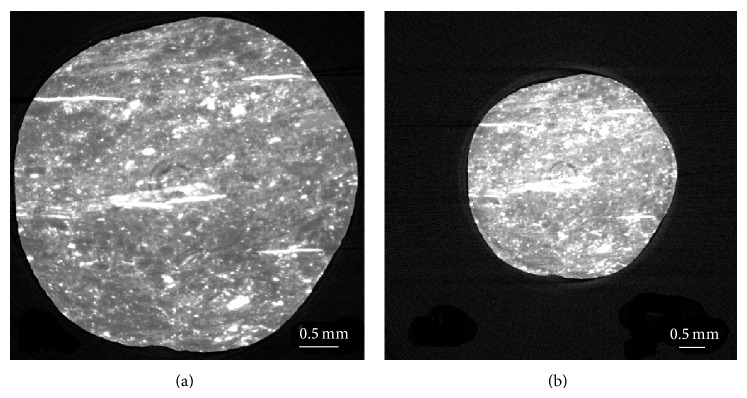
The original reconstructed CT slices at different spatial resolutions: (a) at resolution of 3.7 *μ*m; (b) at resolution of 11.84 *μ*m.
